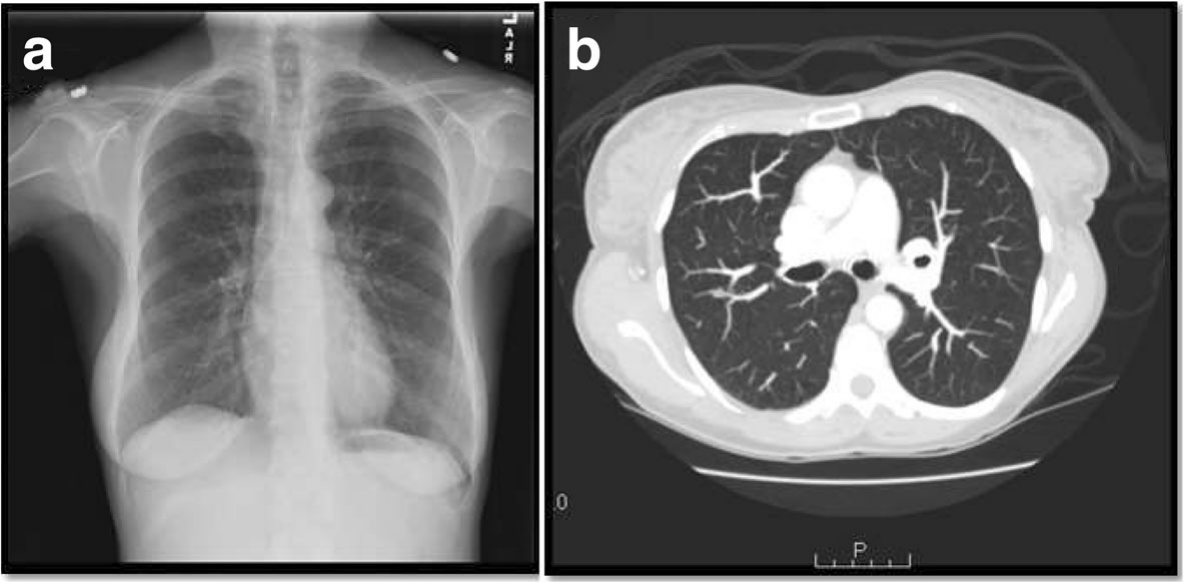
Imaging Results: Normal Chest X-Ray (a) and Chest CT without evidence of intrathoracic lymphadenopathy or pulmonary parenchymal lesions (b)
